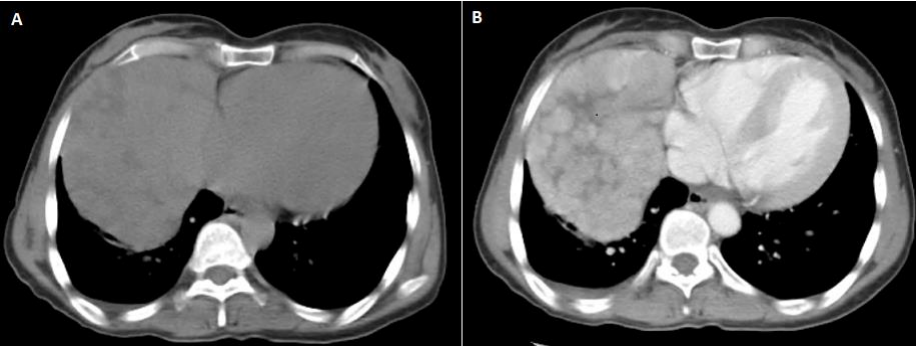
A) non-contrast CT chest and B) contrast-enhanced CT chest: heterogeneously enhancing mass in the right thoracic cavity; it showed a clear limits with polycyclic contours, and it occupies the middle lobe and extends to the upper and lower lobes
